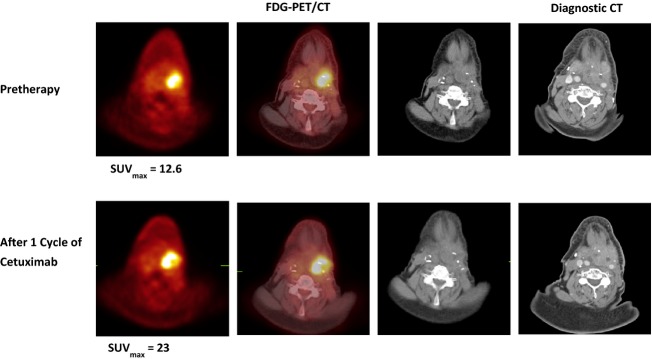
Discordant CT and FDG-PET/CT in a patient (#26) with recurrent left hypopharyngeal squamous cell carcinoma. Transaxial FDG-PET/CT (PET, left; fused PET/CT, middle; CT, right) and contrast-enhanced diagnostic CT images pretherapy (upper row) and after 1 cycle of cetuximab (lower row). The SUV_max_ within the recurrent left hypopharyngeal mass increased from 12.6 pretherapy to 23 after 1 cycle of cetuximab, indicating progression, but the size of the mass was stable on the diagnostic CT scan. SUV_max_, maximum standardized uptake value; FDG-PET/CT, F-18 fluorodeoxyglucose positron emission tomography/computed tomography.
